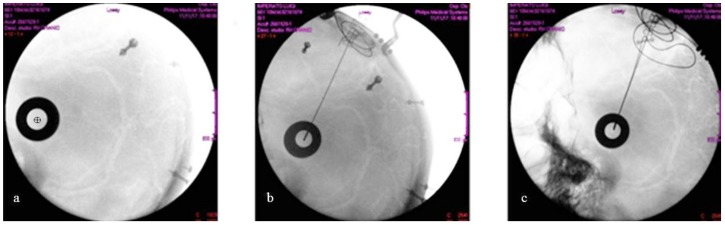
(a) The latero-lateral X-ray image shows the alignment of the radiopaque fiducials. The screws applied to the skull for navigation are also visible. The centre of the circle (black cross) represents the target (i.e. the horizontal entry point). (b) The right electrode hits the target. (c) The final intraoperative control with the two electrodes (latero-lateral X-ray image), after their fixation to the skull with titanium microplates.
